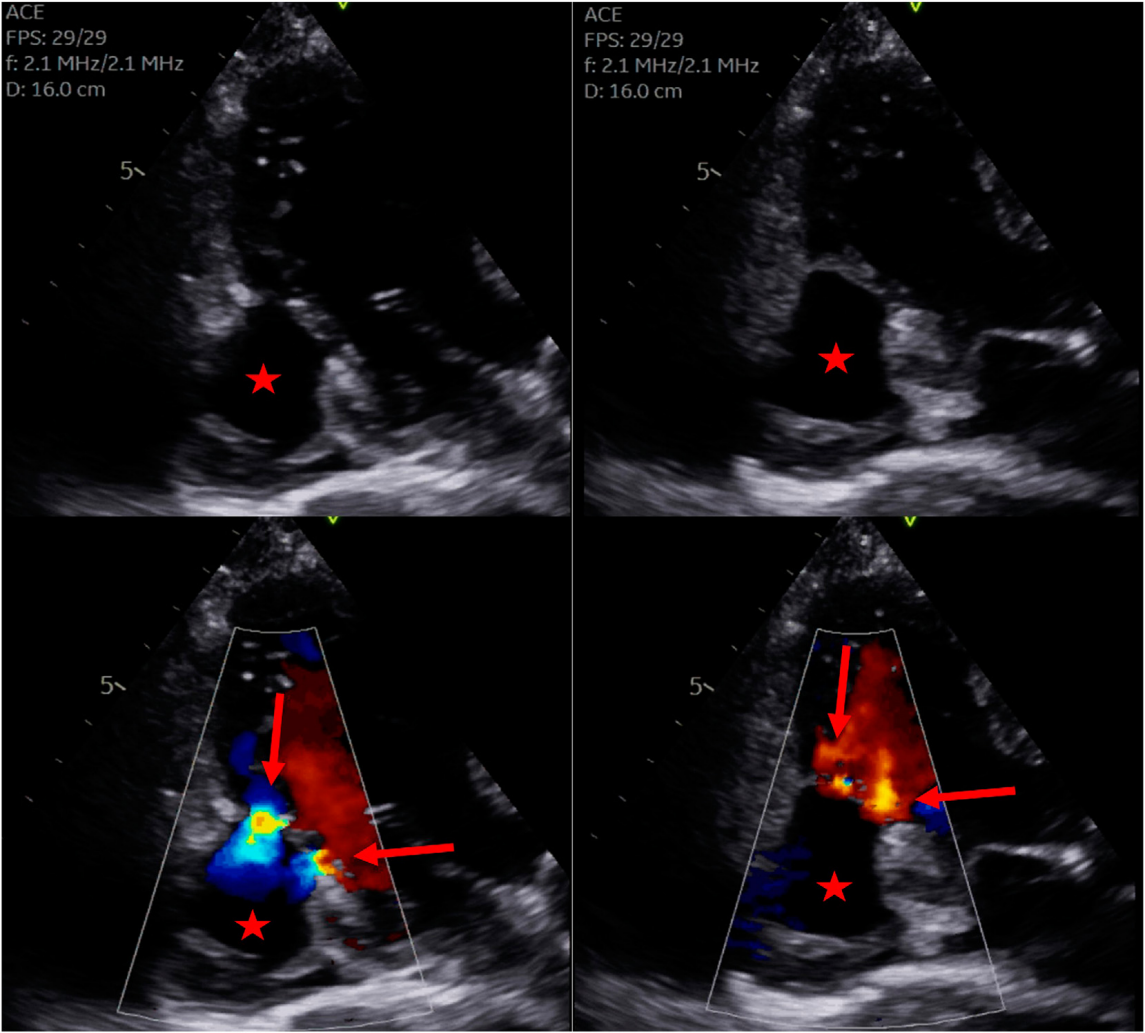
Two-dimensional TTE, apical 3-chamber diastolic (*left*) and systolic (*right*) views, without (*top*) and with (*bottom*) color-flow Doppler, demonstrates a large LVP (*star*) with thin walls in continuity with the basal and mid inferolateral LV myocardium; turbulent to-and-fro flow via 2 orifices (*arrows*) is also demonstrated.
